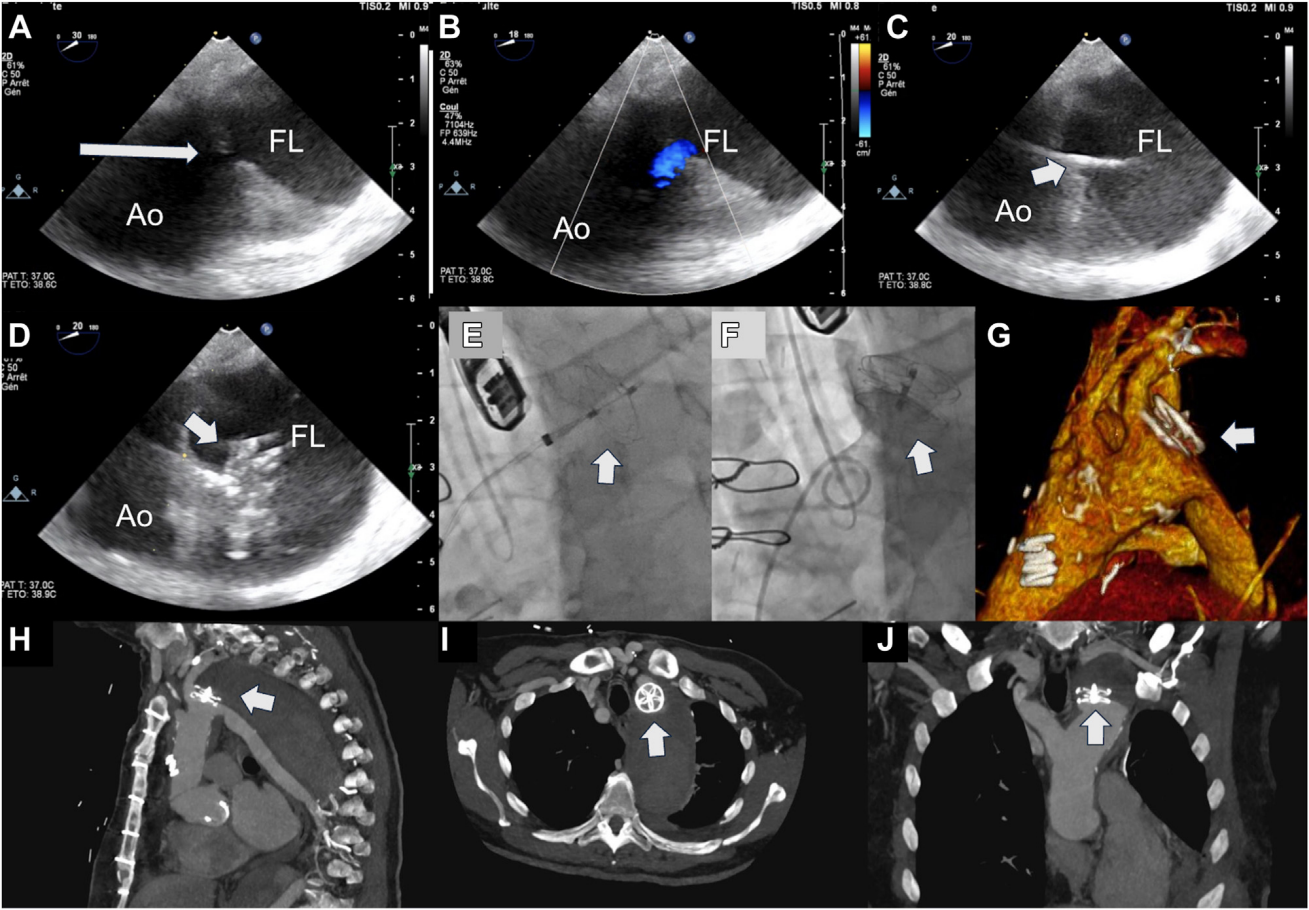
Echocardiographic and fluoroscopic images of the successful GSO device deployment and 4-day postprocedural thoracic-abdominal CT scan multiplanar reconstruction (MPR) and 3D volume rendering images. (A) TEE image showing the re-entry tear in the aortic intima (arrow). (B) TEE image with color Doppler of the re-entry tear, (C) TEE image with the delivery system crossing the re-entry tear (arrowhead). (D and E) Simultaneous TEE and fluoroscopic images showing the device disk opened in the false lumen (arrowhead). (F) Fluoroscopic, (G) 3D volume rendering, and CT scan images in (H) sagittal, (I) transverse, and (J) coronal views showing the device fully deployed and the successful re-entry tear closure (arrowhead). The volume-rendering image (G) reveals the limited protrusion of the GSO occluder disk into the ostium of the left subclavian artery. Ao = aorta; FL = false lumen; TEE = transesophageal echocardiography.
